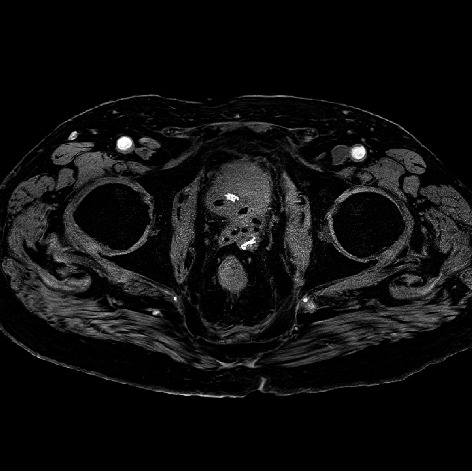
Axial CT of periprostatic collection.
